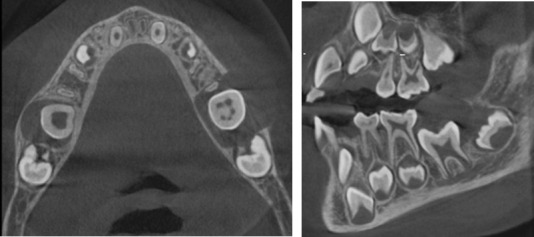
Axial and transaxial view in cone beam computed tomography (CBCT).
